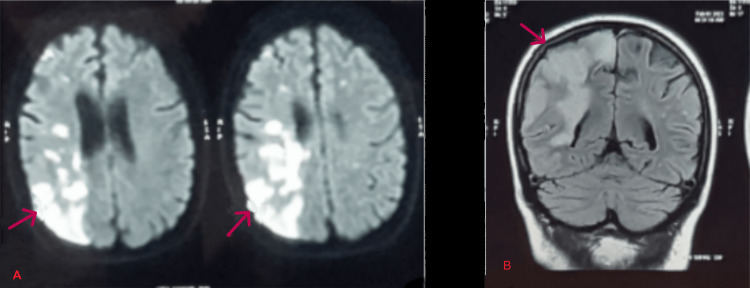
Magnetic resonance imaging of the patient. (A) Restriction on diffusion imaging involving the right posterior frontoparietal cortical-subcortical region and (B) coronal FLAIR image reveal hyperintensity in the right parietal-temporal region. ADC: apparent diffusion coefficient; DW-MRI: diffusion-weighted magnetic resonance imaging; FLAIR: fluid-attenuated inversion recovery
